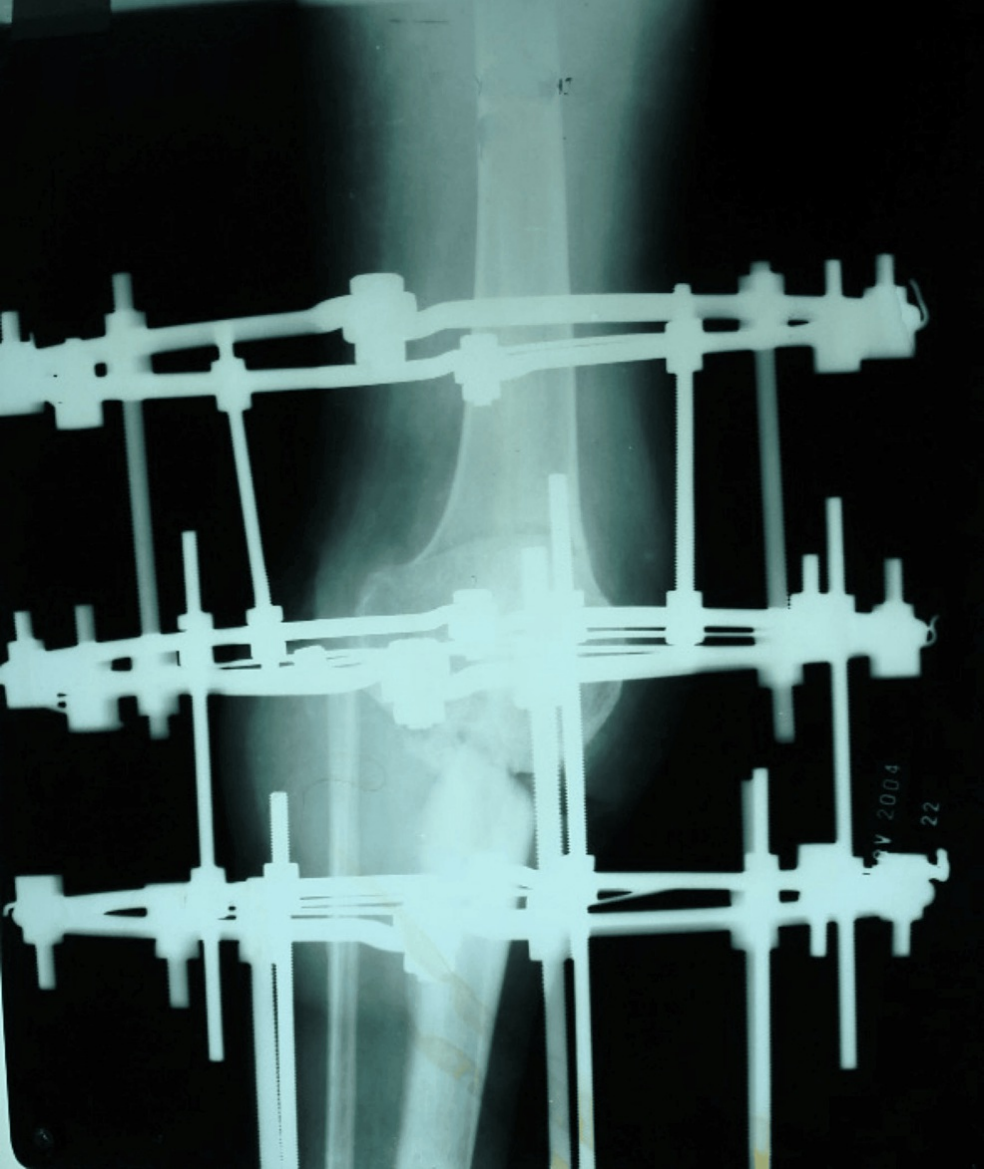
X-ray of a knee joint showing Ilizarov ring fixator with distal femur corticotomy for lengthening.
